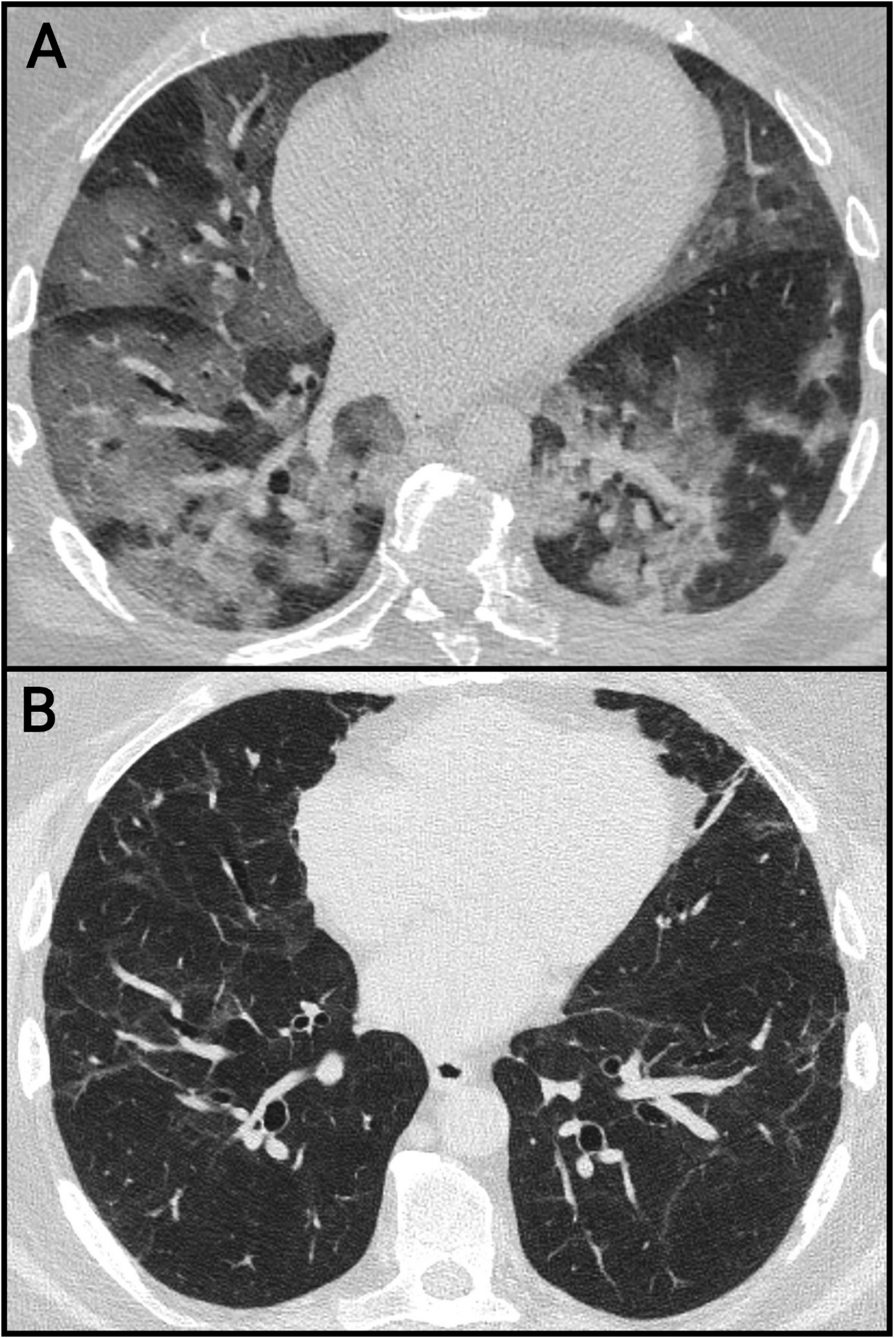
Lung changes on baseline and follow-up chest CT images. The baseline scan (A) shows extensive patchy and confluent ground-glass opacities. The 24-month follow-up scan (B) shows residual banding and mild traction bronchiectasis.
